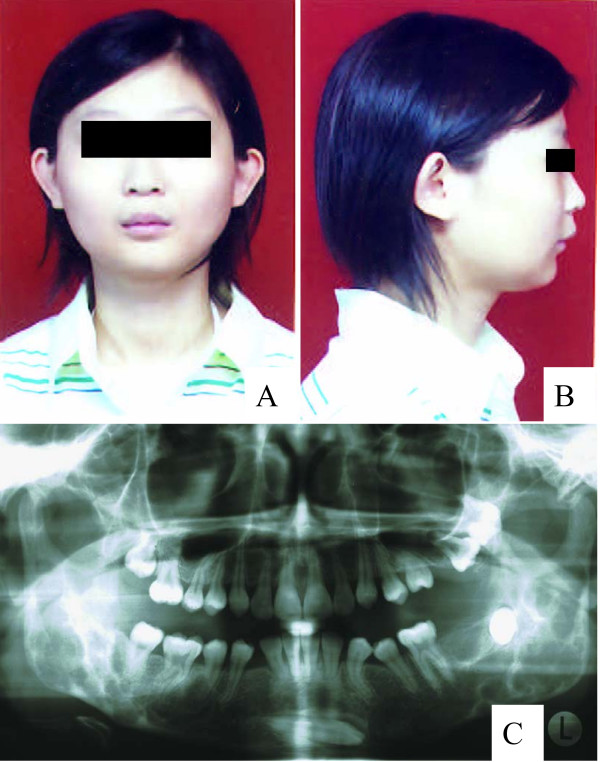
Facial appearances and panoramic radiophotographic findings of affected member III-7.
